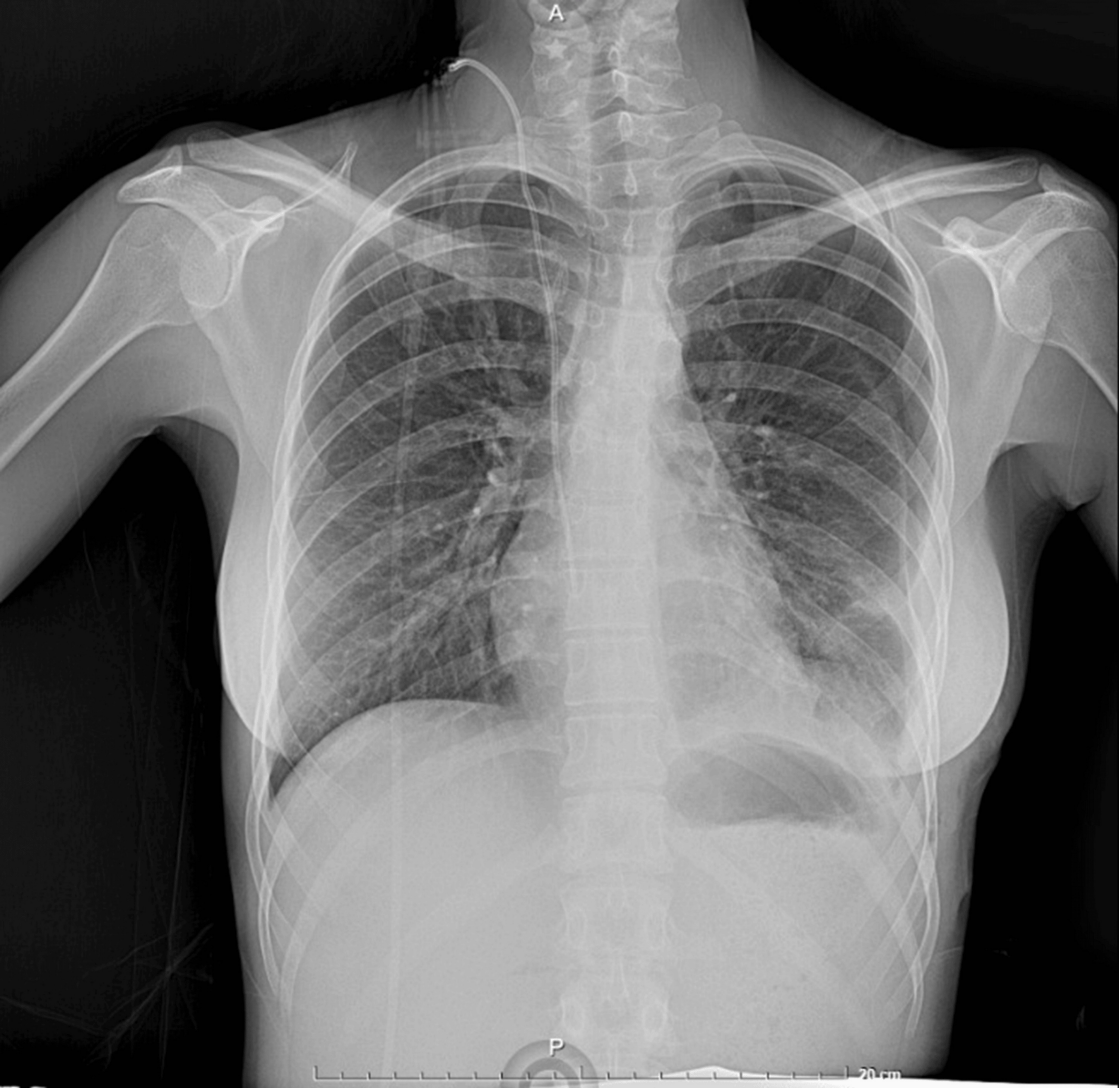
Postoperative chest radiograph showing complete lung re-expansion.
